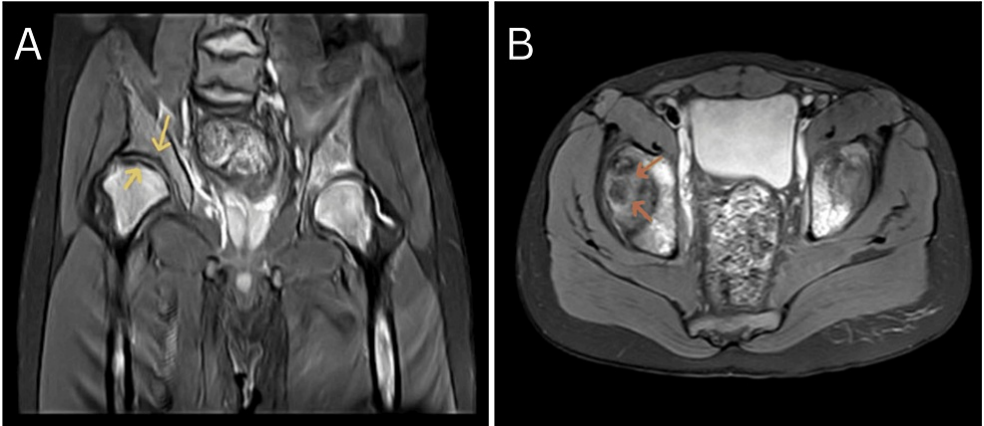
MRI of the bilateral hip joint proton density fat-suppressed image coronal section (A) and axial section (B) showing mild cortical irregularity along the superolateral part of the right femoral head and acetabulum (yellow arrows) and double line sign (orange arrows) suggesting avascular necrosis of the superolateral part of the right femoral head MRI: Magnetic resonance imaging
